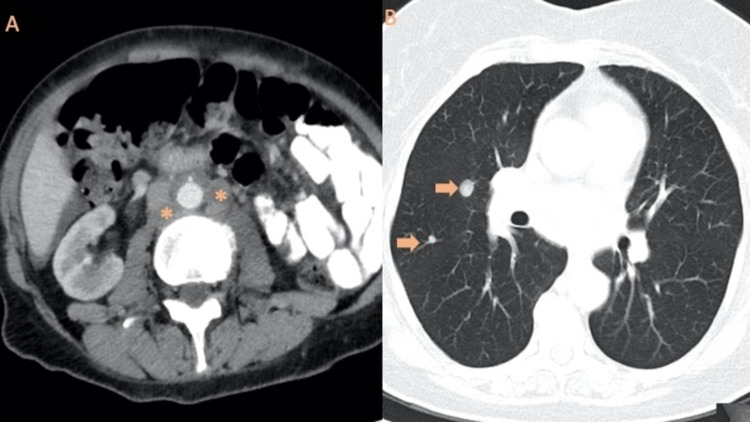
CT findings in two different patients with endometrial cancer A: CT with intravenous contrast in a 54-year-old woman with grade 2 endometrioid adenocarcinoma shows multiple para-aortic lymph nodes metastases (*) which corresponds to FIGO stage IIIC2. B: CT of the thorax in a 67-year-old woman with a clear cell carcinoma of the endometrium demonstrates disease distant spread with lung metastases (arrows).
